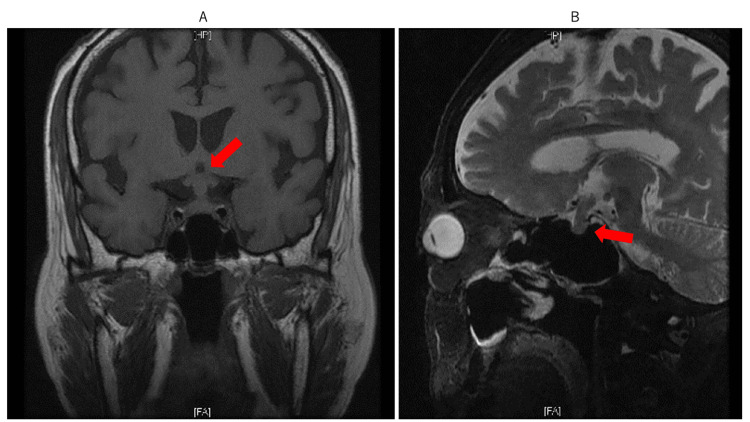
Brain MRI on June 2. A: Coronal section. B: Sagittal section. Note the marked pituitary/pituitary sclerenchyma enlargement (arrows) with associated optic chiasm compression without any abnormal findings in the optic nerve.
